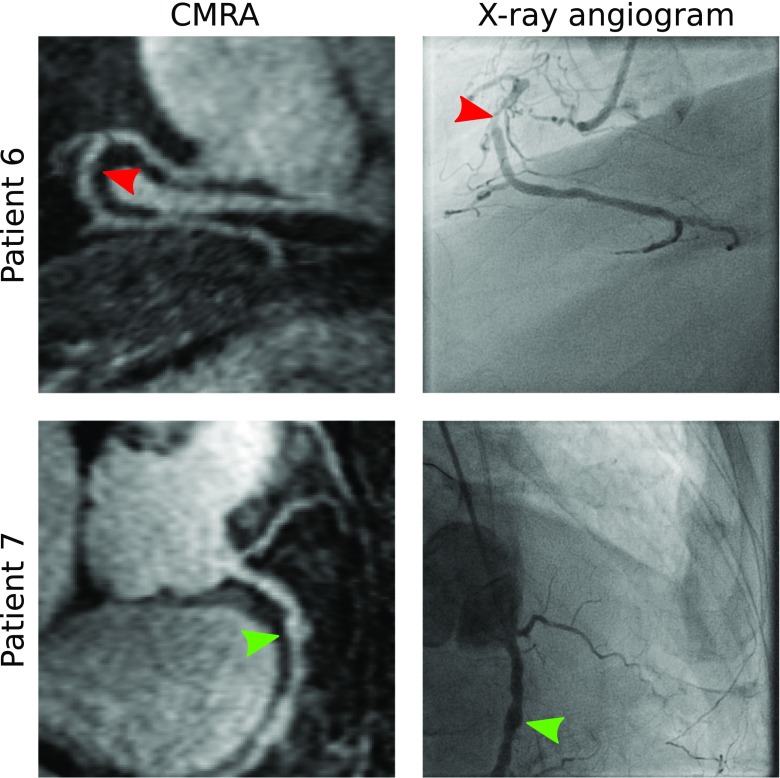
Reformatted CMRA and corresponding X-ray angiogram for two representative patients. *Red arrows*: stenosis in the mid segment of the right coronary artery (RCA); *green arrows*: aneurysm in the proximal segment of the RCA
